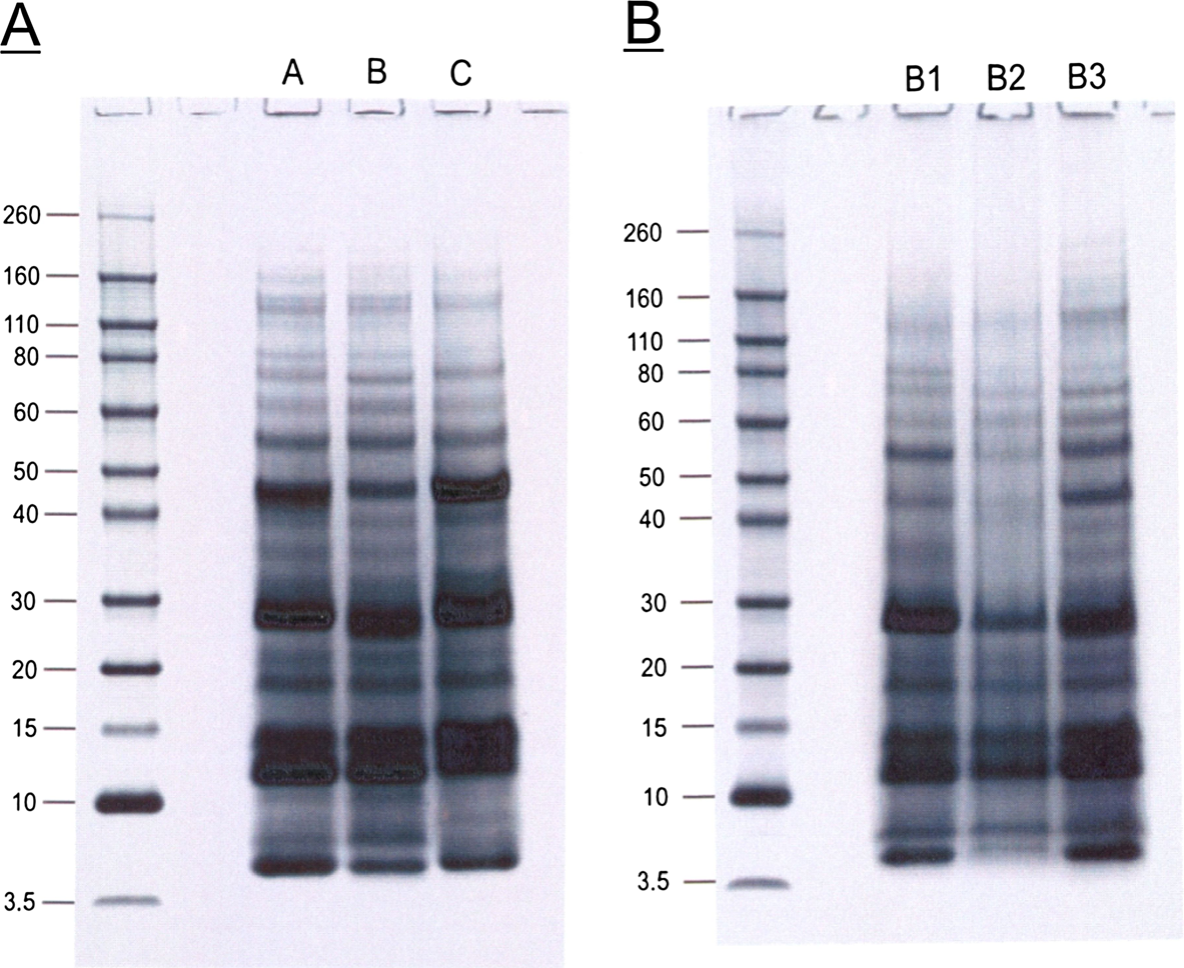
PAGE comparison of acid-soluble matrices from shells. Molecular weight markers are indicated at the left. Each lane was loaded with 200 μg of matrix in a volume of 30 μl. A, matrices of shells cleaned with different sodium hypochlorite protocols. Lane A, 2 h hypochlorite at room temperature; lane B, 2 h hypochlorite with 2 x 5 min ultrasound treatment at the start of each hour; lane C, cleaned with hypochlorite for 24 h with 2 x 5 min ultrasound bursts as before and one after 24 h. B, matrices of different shells, all cleaned with hypochlorite according to protocol B (2 h hypochlorite, 2 x 5 min ultrasound).
